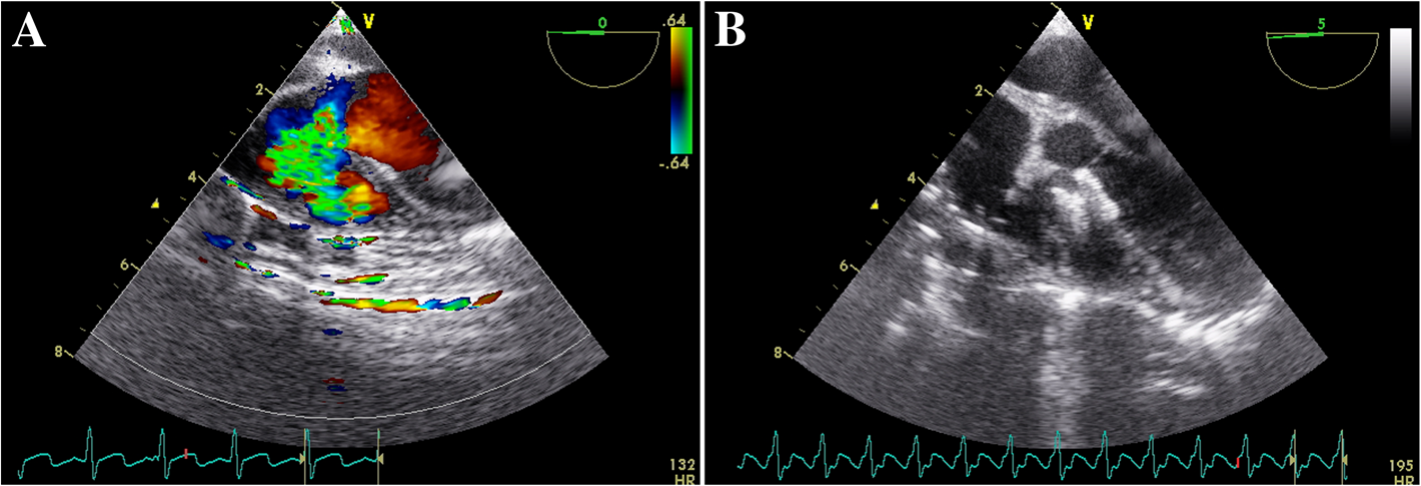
A Color Doppler imaging of the TEE four-chamber view showing a VSD. B Occluder was deployed with left and right disc in right position.
